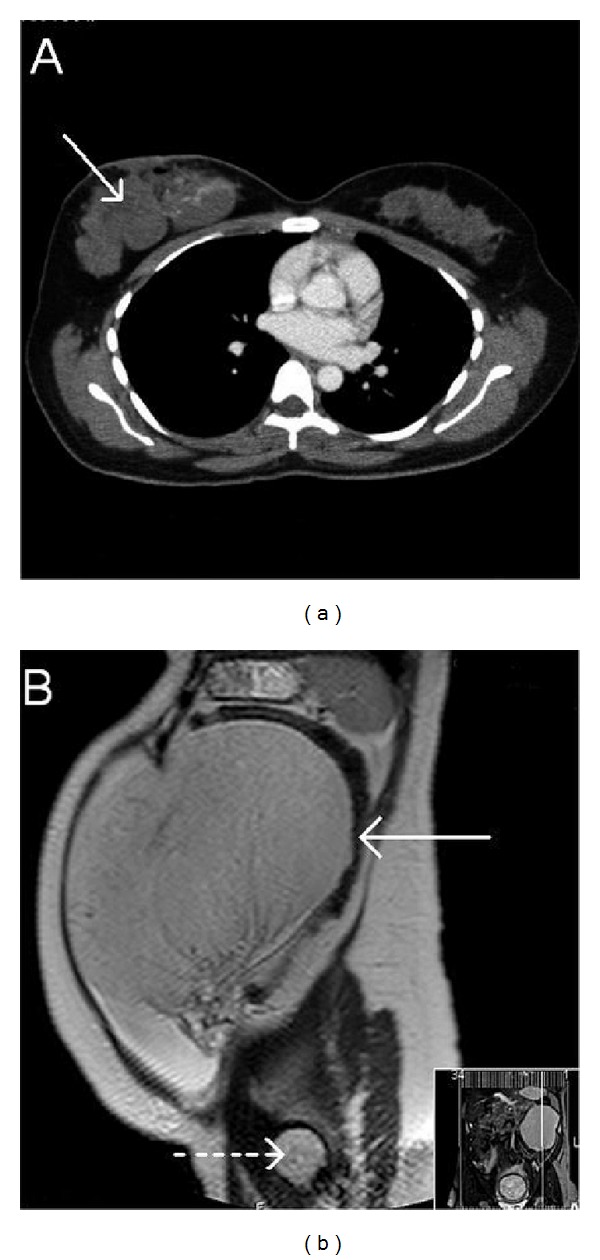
(a) Computed tomography (CT) scan show multifocal masses in the deep parenchyma of the right breast (arrowed); the largest measuring 4.6 cm in maximum diameter. (b) Magnetic resonance imaging (MRI) scan showed a large 19 × 17 × 8.3 cm smooth, circumscribed homogeneous solid mass in the left upper quadrant (arrowed), which was seen to displace the gravid uterine fundus to the left of the midline (dotted arrow), without mural invasion.
